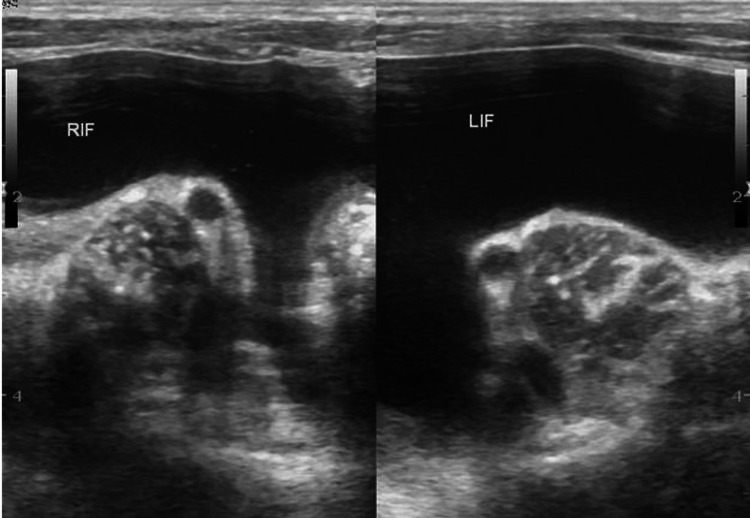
High-resolution ultrasonography image of the pelvis demonstrating fluid collection in the right and left iliac fossae. The margins of the urinary bladder were not separately visualized. RIF: right iliac fossa, LIF: left iliac fossa.
